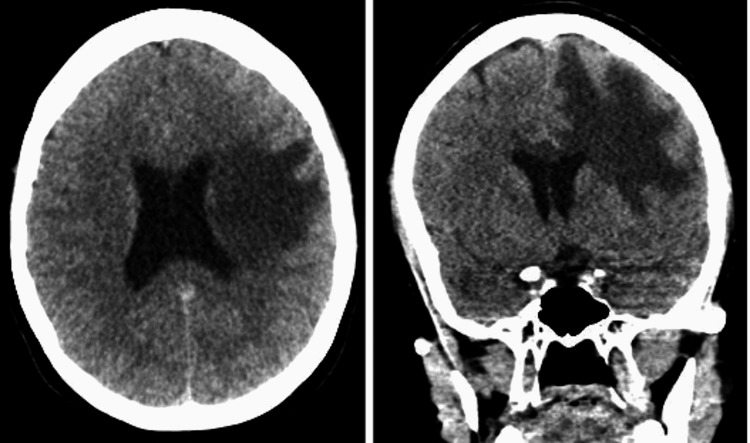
Simple cranial CT scan February 07, 2021 Axial (left) and coronal (right) sections, periventricular edema is observed in the left frontal region, with digitiform characteristics without displacement of the midline structures, without occupational lesion.
